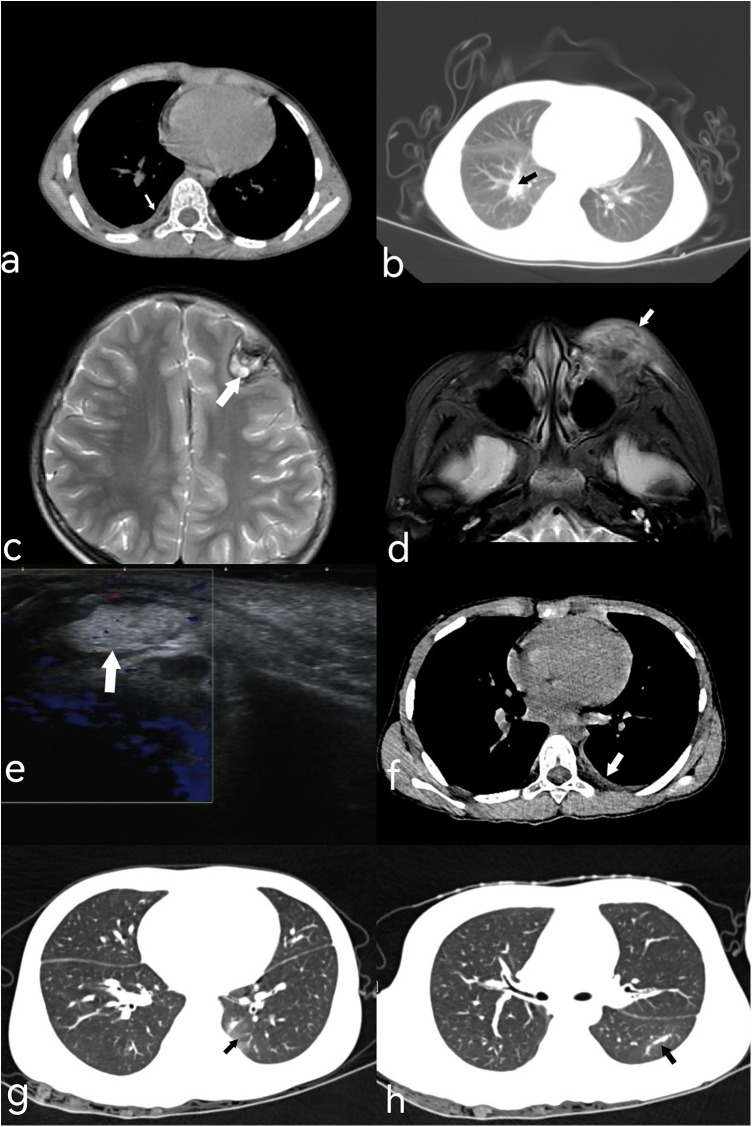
The imaging examination images of the twin sisters. (a) Chest CT (elder twin): Small right pleural effusion (white arrow). (b) Chest CT (elder twin): Patchy hyperdense opacities in right lower lobe (black arrow). (c) Brain MRI (3.0T, T2W-TSE, elder twin): Flaky T2 hyperintensity in left frontal lobe with circumferential marginal hypointensity (white arrow). (d) Orbital MRI (3.0T, OCor STIR, elder twin): Left periorbital soft tissue swelling with T2 hyperintensity (white arrow). (e) Ocular color Doppler ultrasound (elder twin): Irregular hyperechoic mass under left eyelid (white arrow). (f) Low-dose chest CT (younger twin): Slight left pleural thickening and small pleural effusion. (g,h) Chest CT (younger twin): Lesions in dorsal/posterior basal segments of left lower lobe (black arrow).
